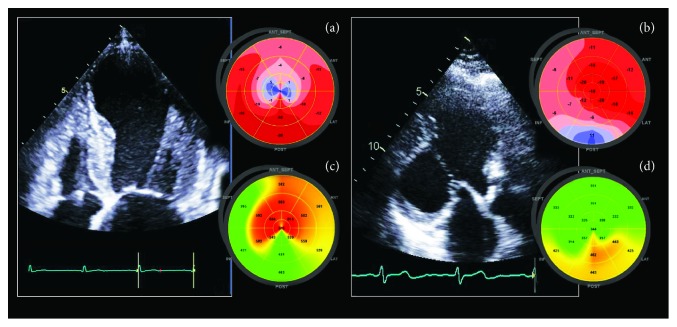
Echocardiographic assessment of postinfarction left ventricular remodeling through two-dimensional speckle-tracking echocardiography longitudinal strain bull's-eye maps in one patient with anterior myocardial infarction (a) and in another with inferior myocardial infarction (b). Red/blue bull's-eye maps (a, b) clearly identify regional alterations of longitudinal strain in the segments more affected by myocardial infarction (thin and dilated), whereas green/yellow bull's-eye maps (c, d) describe delayed activation (yellow and red areas) in the same segments, which can determine mechanical dispersion and increased risk of arrhythmias.
